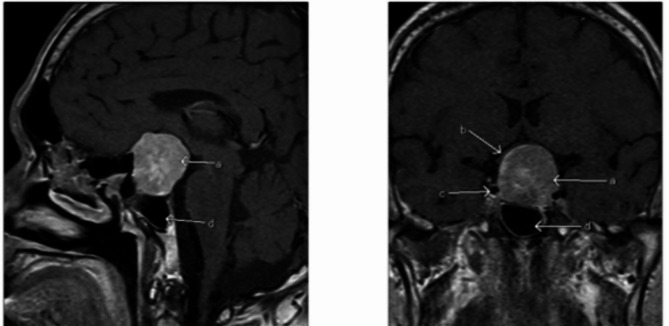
Sagittal and coronal views of Magnetic Resonance Imaging (MRI) of the pituitary gland at the initial presentation showing large enhancing sellar and suprasellar mass lesion (a), compressed and elevated optic chiasm (b), and tumor contacting cavernous portion of the internal carotid artery (c) and sphenoid sinus (d)
